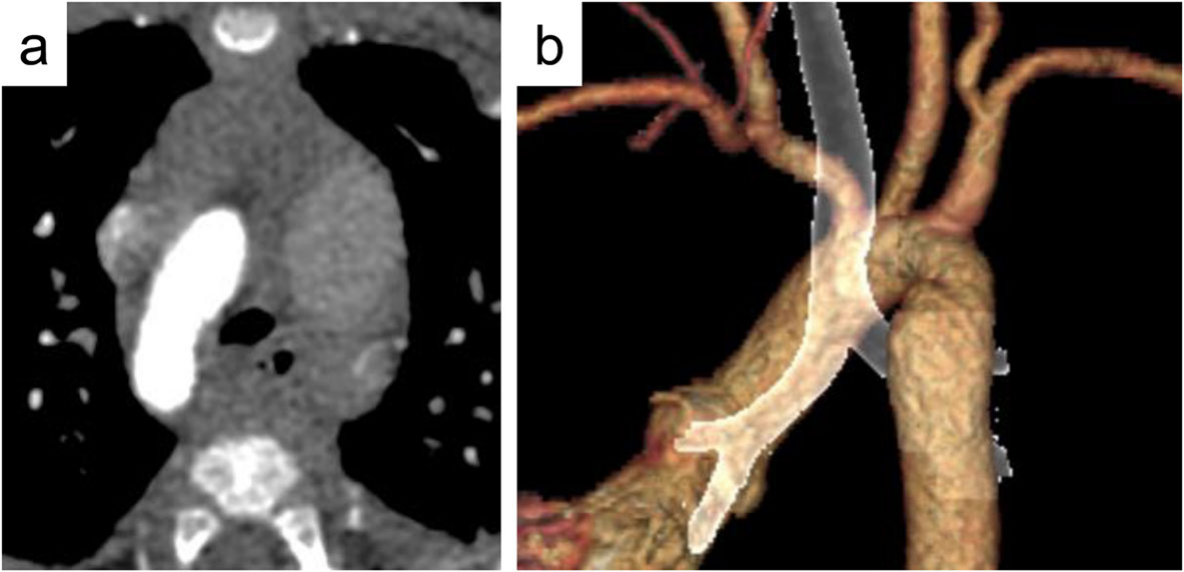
Chest CT at postoperative status describing the release of tracheal compression. a Horizontal image in chest CT. b 3D image from the left back in chest CT
